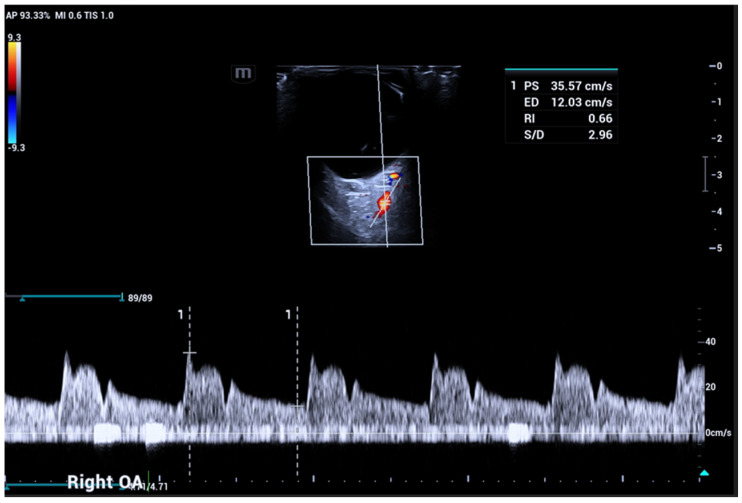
Ophthalmic artery (OA) courses in the superomedial part of the orbit and is usually depicted in red, with a Doppler signal characterized by a peak systolic velocity (PSV) variable from 30 to 60 cm/s: angle correction is essential to obtain reliable velocity measurements.
